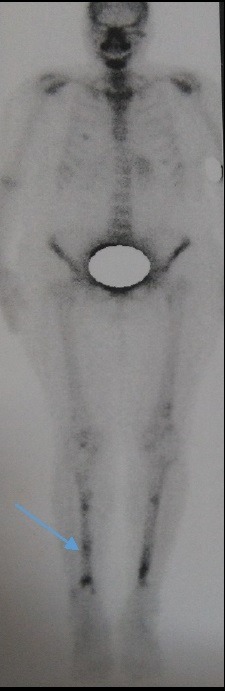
Tc99m-methyl-diphosphonate scintigraphy showing symmetric uptake of the radiotracer in the tibial diaphysis and metaphysis regions
